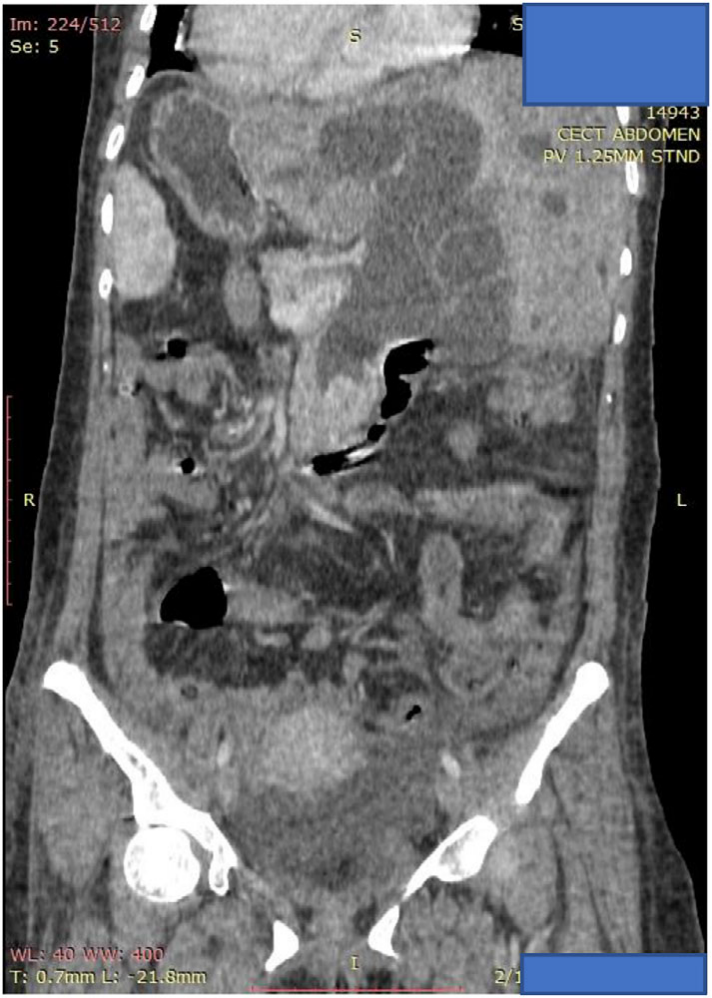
CT imaging revealed choledocholithiasis with upstream dilatation of the biliary tree
